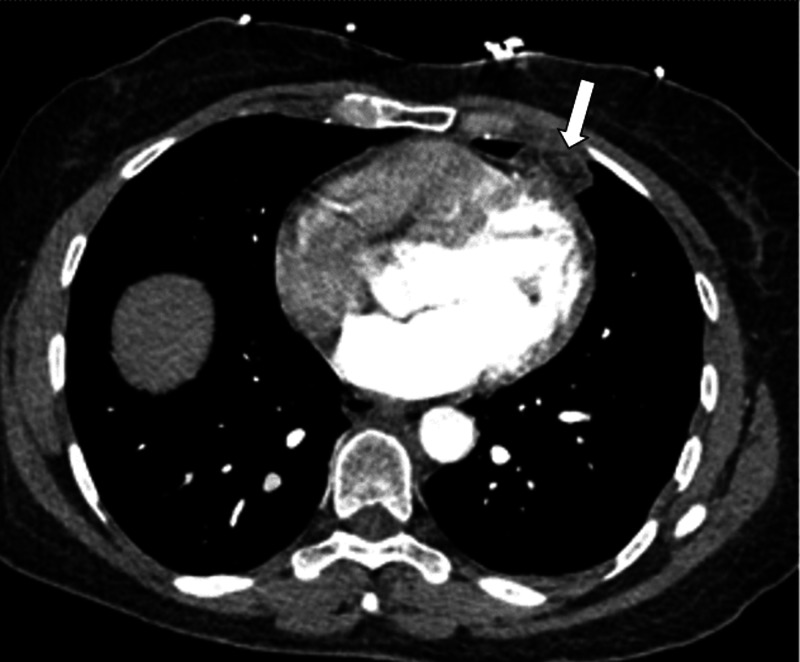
Computed tomography showing epicardial fat necrosis as ovoid-shape mass with a rim, central high attenuation focus, and surrounding soft tissue stranding as indicated by an arrow. Image provided by the Beaumont Hospital, Royal Oak with permission for use.
